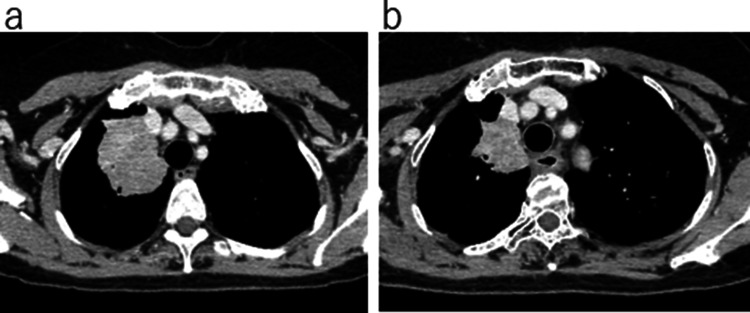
a CT, and PET-CT images before induction chemoradiation therapy. Chest CT scan showed a 56 mm right upper lobe mass shadow; PET-CT showed FDG accumulation in a lung mass and #4R lymph node. b Images after induction chemoradiation therapy
